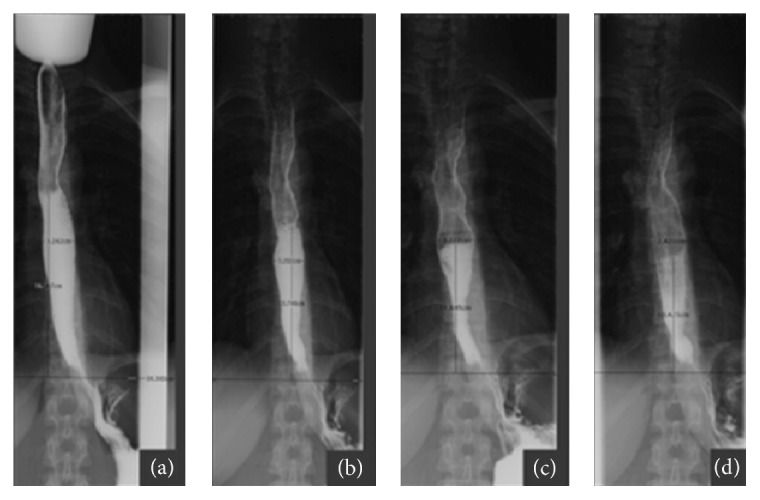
X-rays show the height of barium column 0, 1, 2, and 5 minutes after barium oral administration.
